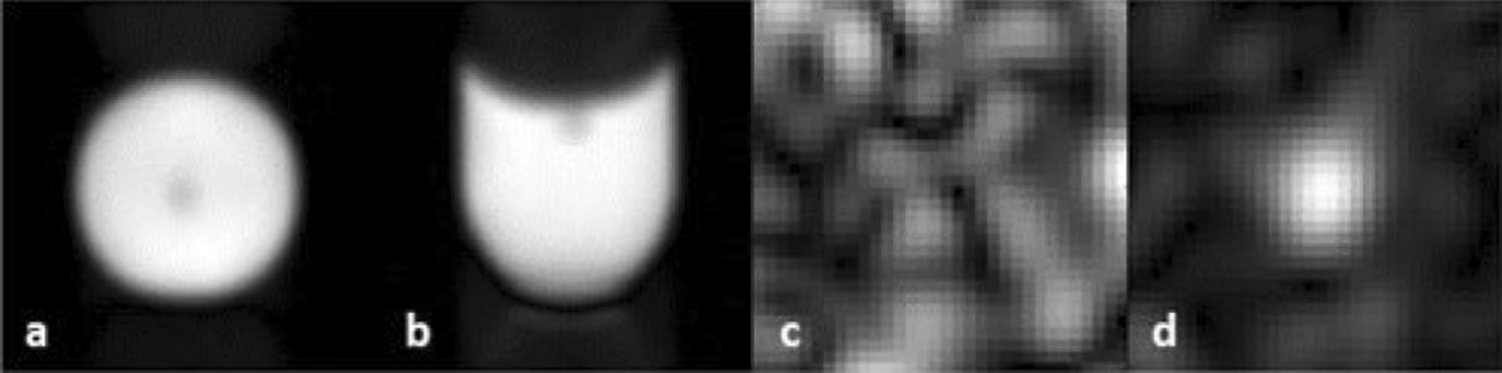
Two-dimensional Turbo spin echo (2D-TSE) ^1^H and ^19^F MRI. (a, b) High-resolution ^1^H images of the sample (one average, matrix size 32 × 32, TE 6 ms, phase-oversampling (PhOS) factor 8, read-oversampling factor 2, field-of-view (FoV) 15 × 15 mm^2^, one slice covering the whole sample, data fourfold zero-filled). The central signal decrease reflects the position of the fiber. Due to the two-dimensional acquisition technique, the image represents a projection along the direction orthogonal to the displayed plane. a: ^1^H image, axial view, b: ^1^H image, coronal view. (c, d) ^19^F images without (c) and after 4 s of illumination (d) (one average, axial view, matrix size 8 × 8, FoV 15 × 15 mm^2^, one slice covering the whole sample, PhOS 4, Turbo factor (TF) 32, zero-filling factor 4). Without illumination, only noise can be seen, while after illumination the signal is clearly visible in the center of the image.
